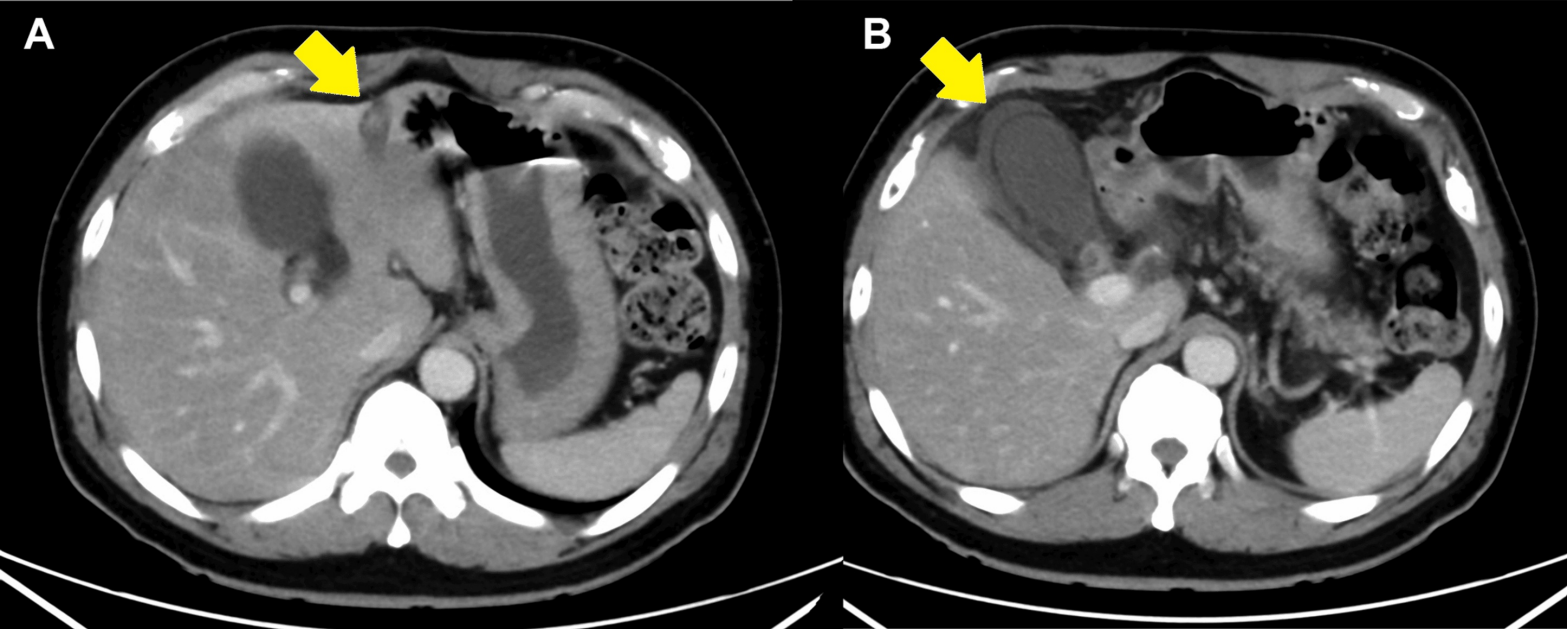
Computed tomography scan with late phase contrast on the third day. (A) Portal vein thrombosis in the left branch and no partial enhancement in the liver were observed. (B) Gallbladder infarction with no enhancement nor thickening of the gallbladder wall was observed.
